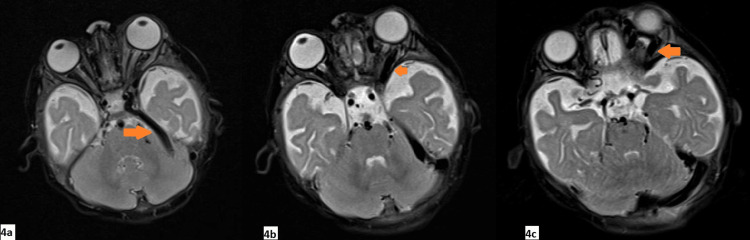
Axial (4a, 4b, and 4c) T2-weighted MR images showed dilatation of the left superior petrosal sinus (4a) and left superior ophthalmic vein (4b and 4c)
